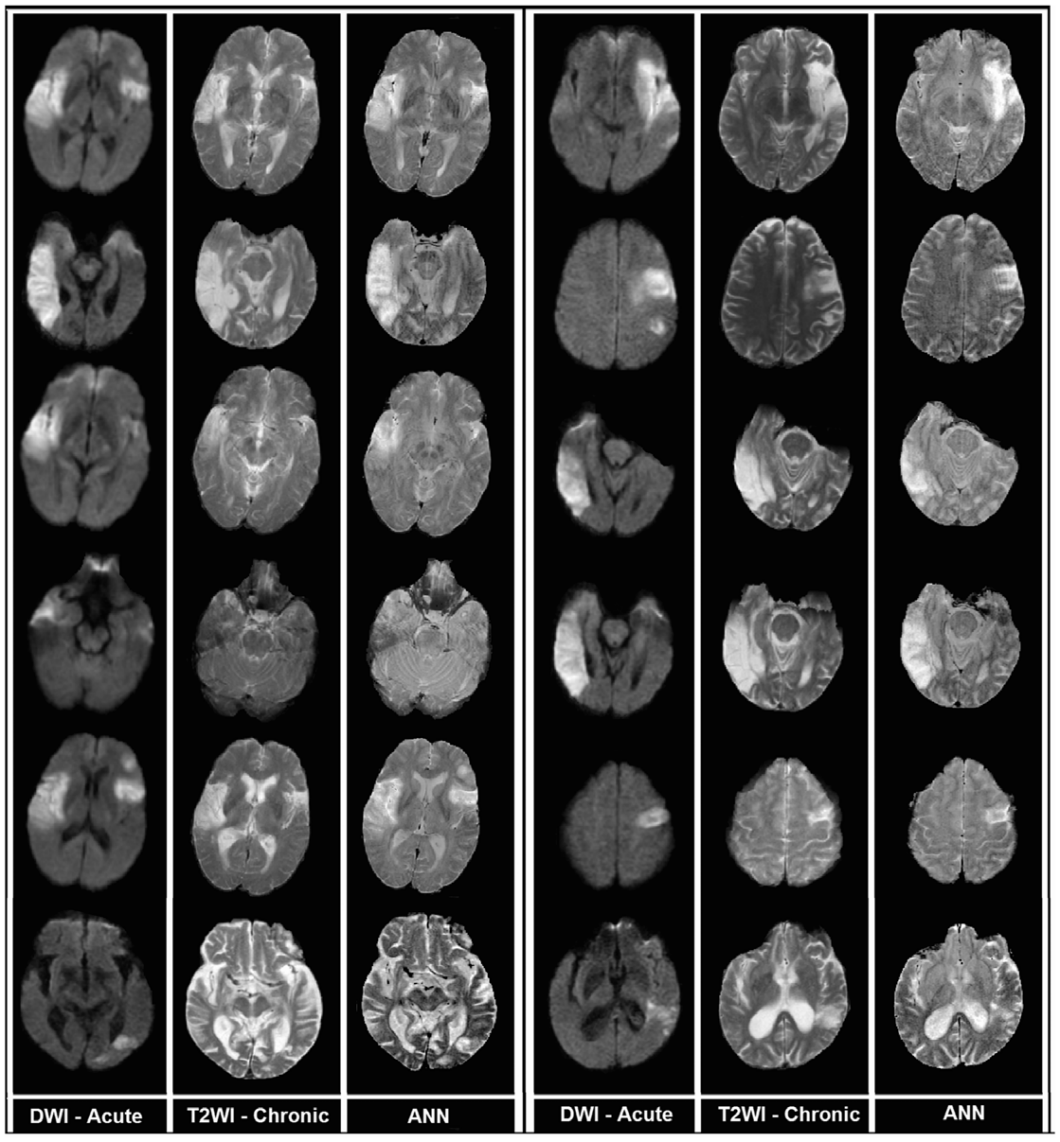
Examples of ANN-produced images in 12 exemplary MRI slices from 9 patients. Left column: DWI in acute study. Middle: outcome T2WI. Right: ANN predicted outcome from acute image set. The last slices in both columns present the least reliable prediction of the ANN.
